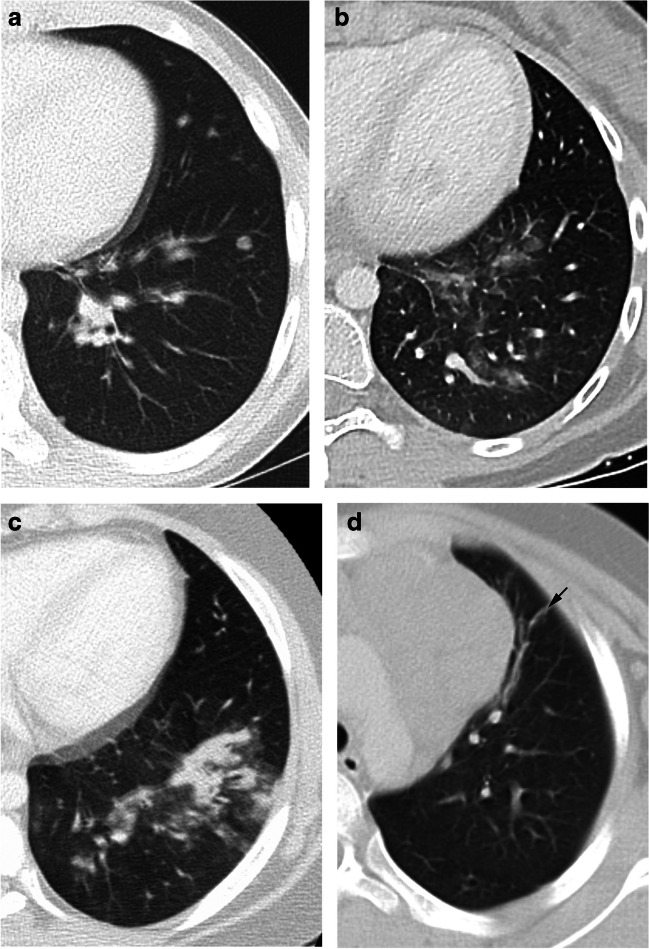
Axial computed tomography scans on lung windows show the different morphological patterns of lung lesions that developed between initial staging and early response assessmen in patients with Hodgkin lymphoma. a A nodule in a 14-year-old boy. b Ground-glass opacity in a 14-year-old girl. c Consolidation in a 12-year-old girl. d Parenchymal band (*arrow*) in an 8-year-old girl
